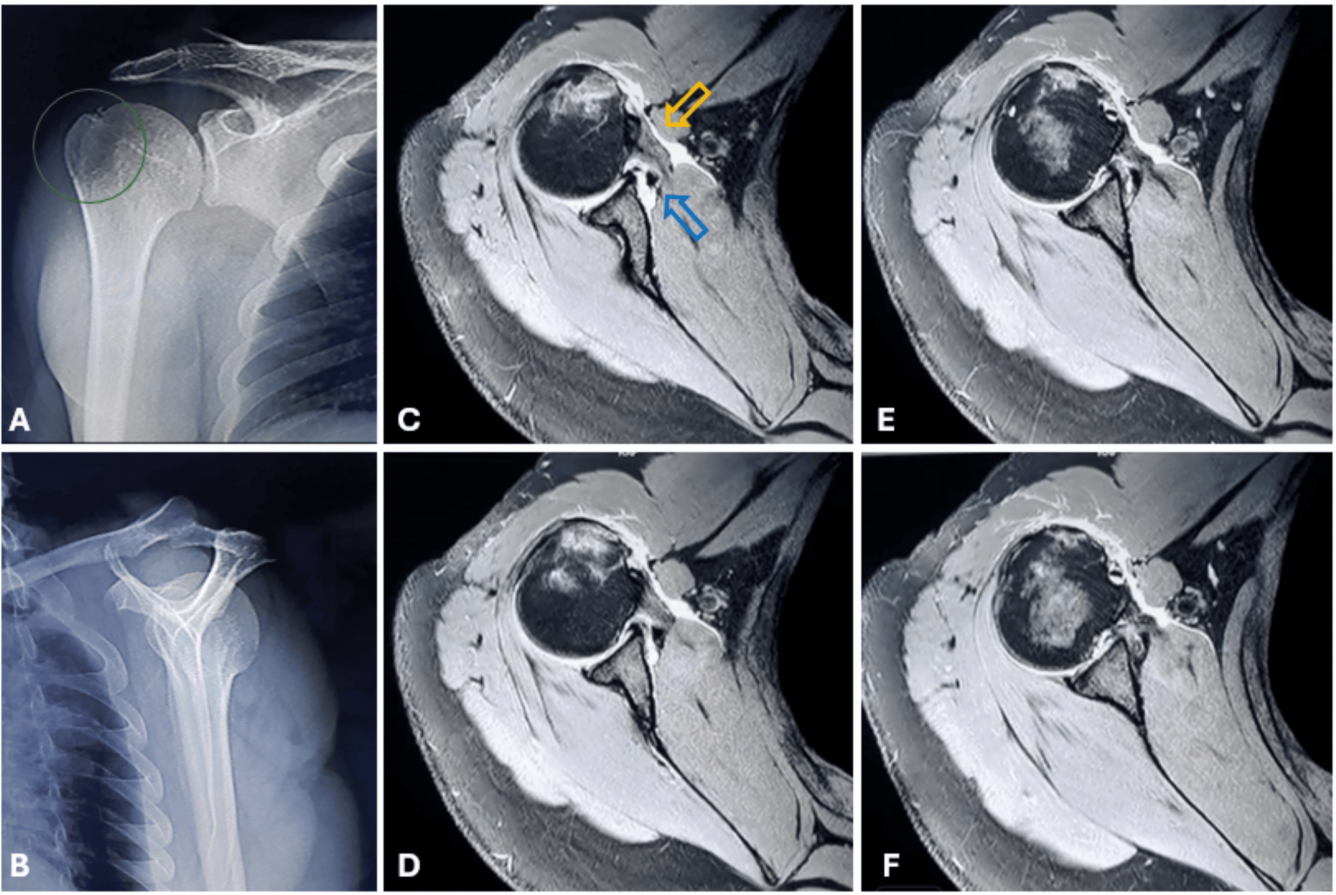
Imaging studies of a patient with a right greater tuberosity fracture after falling from a height and hanging in mid-air by his right shoulder. A and B are direct radiographs, and C, D, E, and F are consecutive axial T2-weighted MRI images. Figure 1A: True anteroposterior radiograph showing the right shoulder with a non-displaced greater tuberosity fracture (green circle). Figure 1B: Scapular Y radiograph highlighting normal alignment. Figures 1C-1F: Consecutive T2-weighted MRI sequences in axial sections displaying partial subscapularis tear (orange arrow), suspected ALPSA lesion (blue arrow), bone marrow edema, and widespread periarticular effusion.
